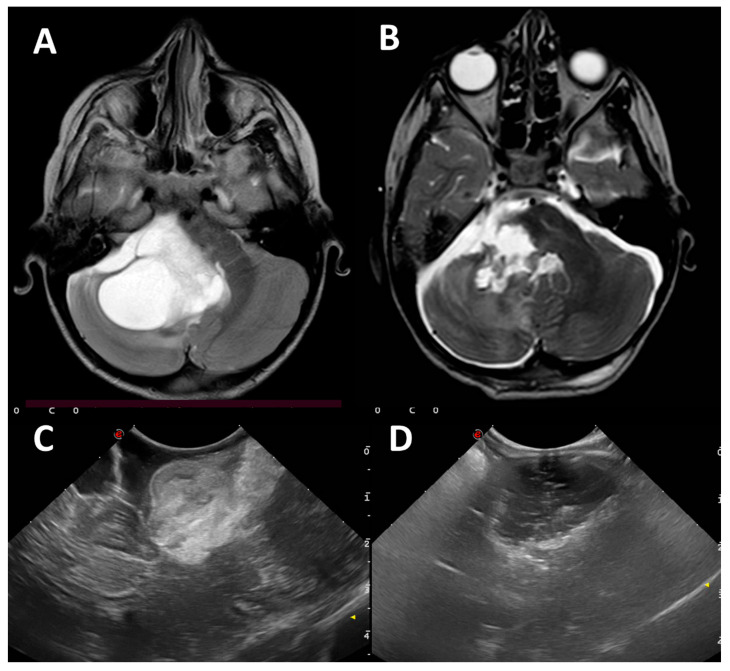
T2 axial MRI showing the preoperative (A) and postoperative picture (B) of a low grade glioma (9-year-old girl) arising from the brainstem and extending into the right CPA. IOUS clearly show the tumor (C) and its GTR (D).
